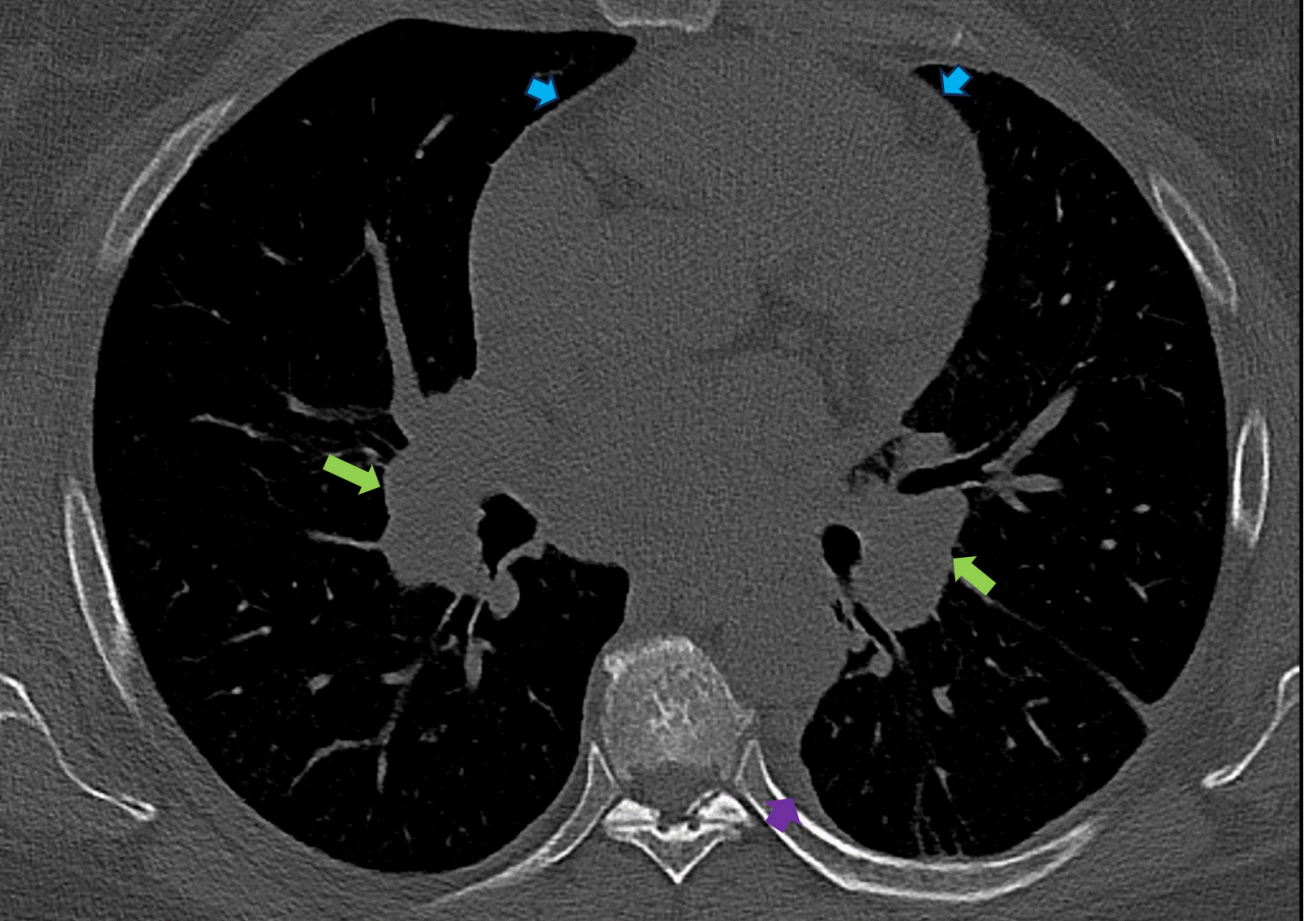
CT chest 8 months after treatment. The blue arrows indicate significant interval improvement in the pericardial effusion. The purple arrows highlight the near resolution of the left‐sided pleural effusion. The green arrows show the persistence of bulky hilar lymphadenopathy bilaterally.
